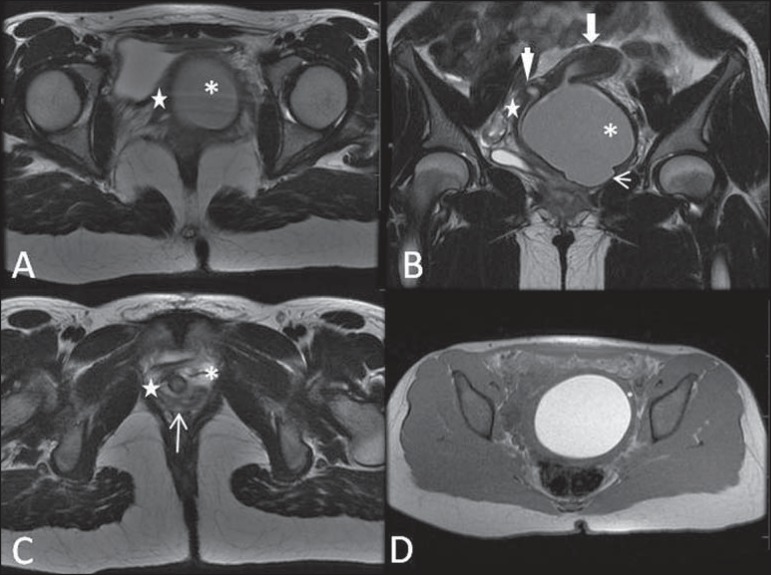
Uterus didelphys, longitudinal and transverse vaginal septa. MRI T2- weighted
